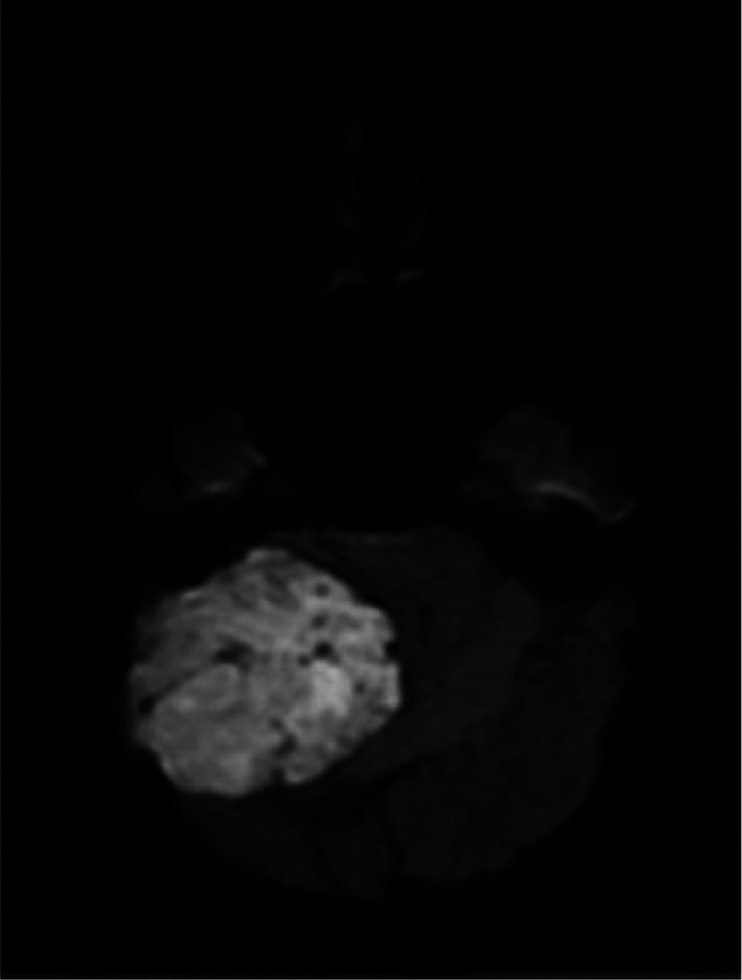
Restricted diffusion area on DWI.
